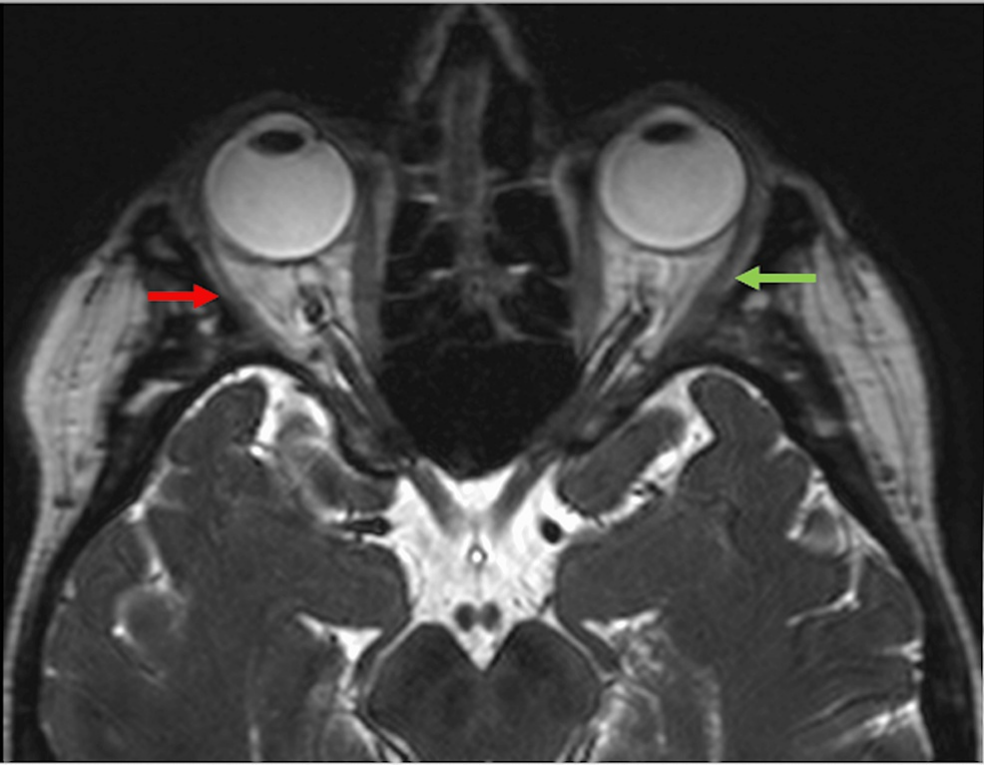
Axial 3D T2-weighted image of a bilateral orbit. The image demonstrates atrophy of the right lateral rectus muscle (red arrow) compared to the normal left lateral rectus muscle (green arrow).
